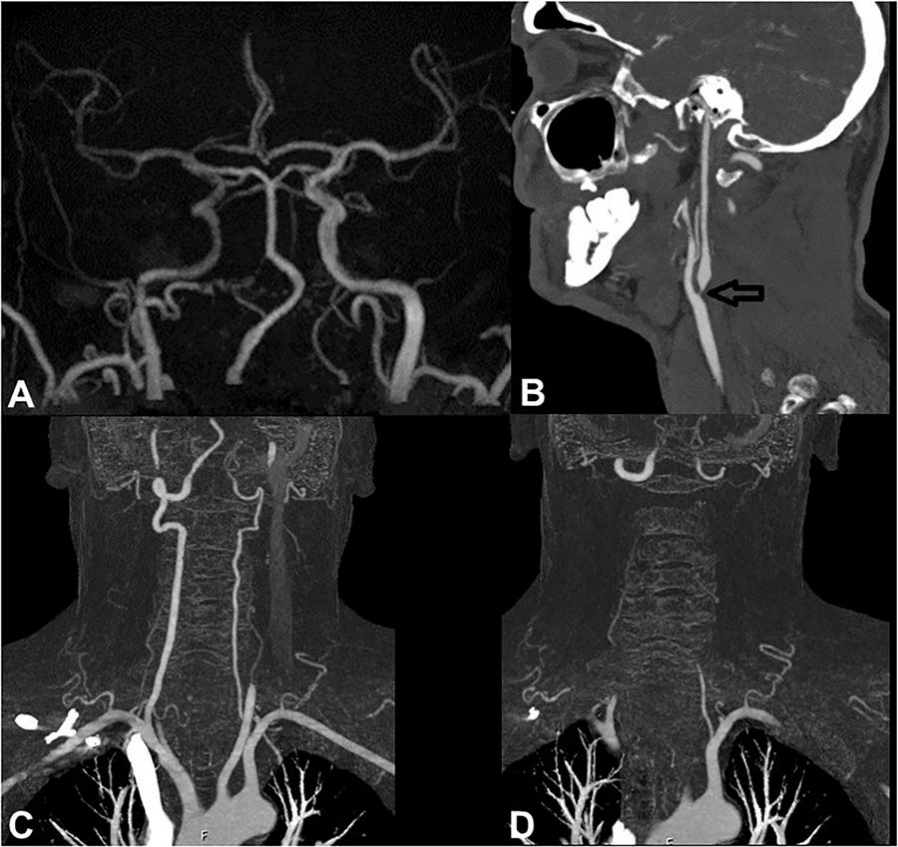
(A) Cerebral MRI showing on time of flight sequence showed a right intracranial ICA of small diameter and low-intensity signal compared to the left indicating a low blood flow. (B–D) CTA showing a sub-occlusive right carotid bulb atherosclerotic stenosis (black arrow), the absence of abnormality of the subclavian arteries and the origin of the vertebral arteries.
